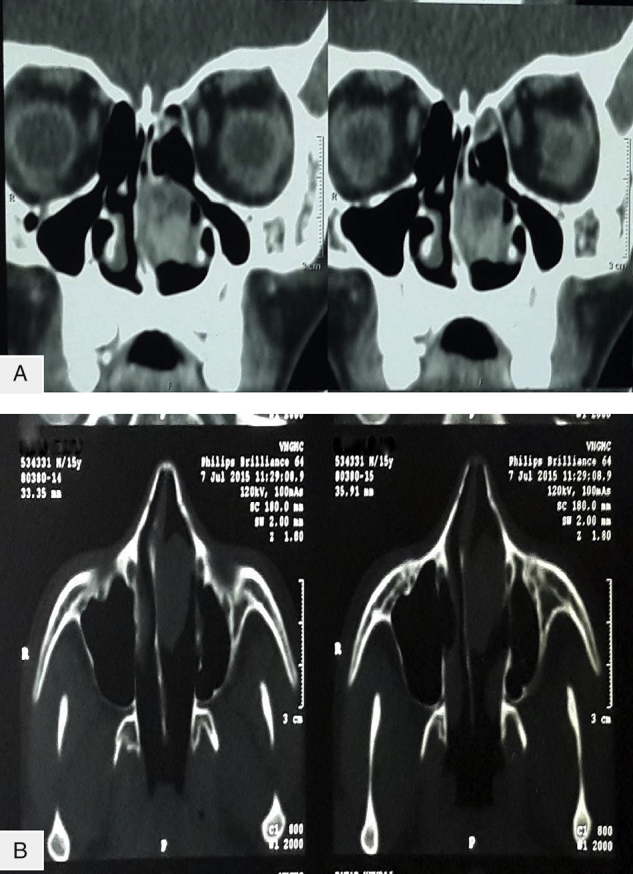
Preoperative CT scans (A) coronal and (B) axial views showing a soft tissue density lesion in left nasal cavity with heterogenous contrast enhancement.
